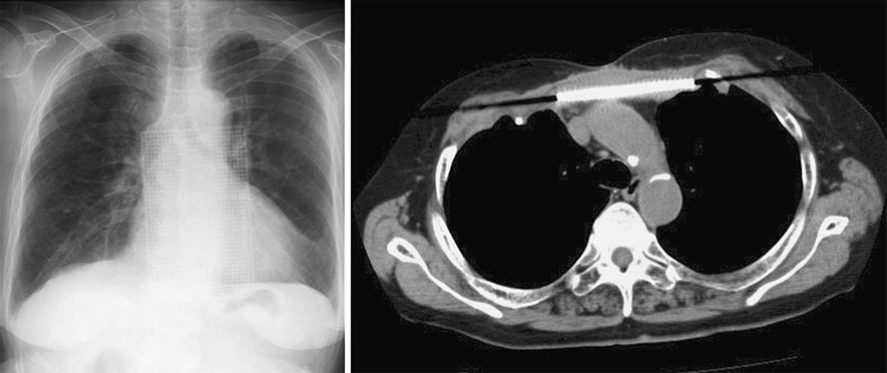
Postoperative chest X-ray and computed tomography scans showing the titanium plates secured to the manubrium and ribs
